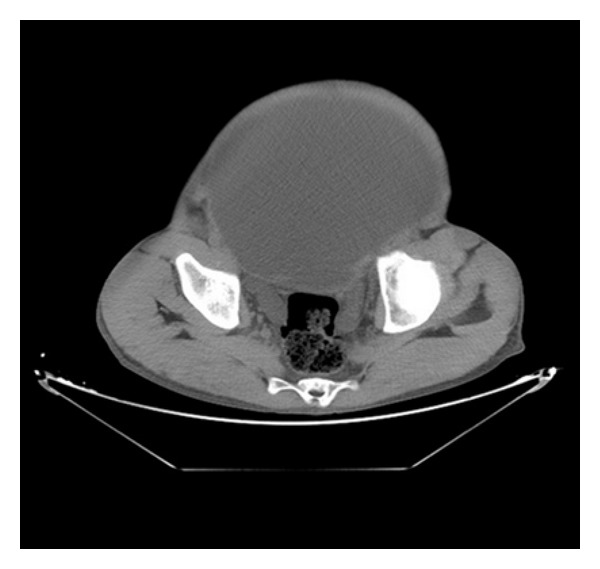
Caudal pelvic CT scan showing a huge urinary bladder.
